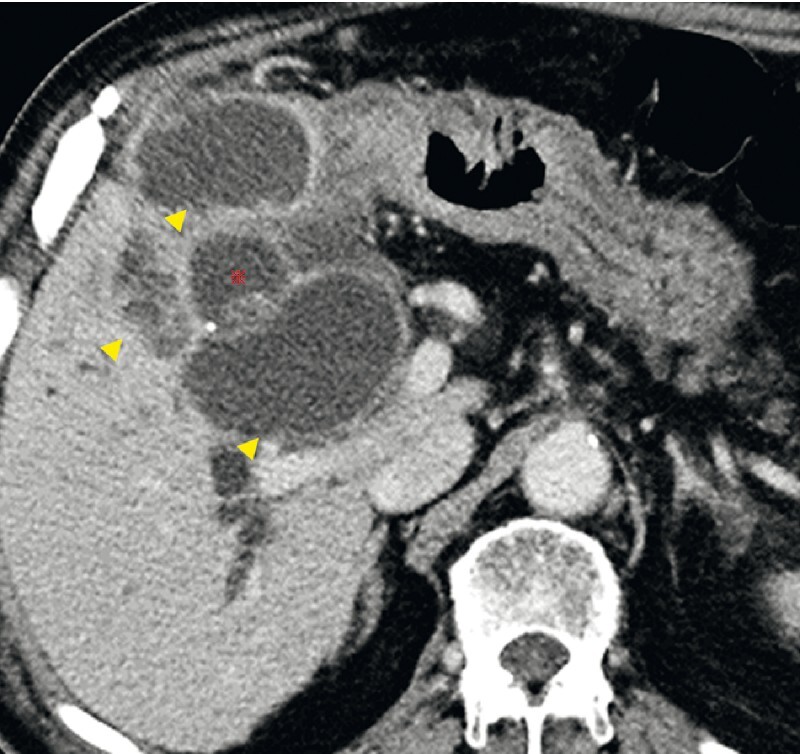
Computed tomography shows the multiple abscess (yellow arrowheads) around the gallbladder (red asterisk).
